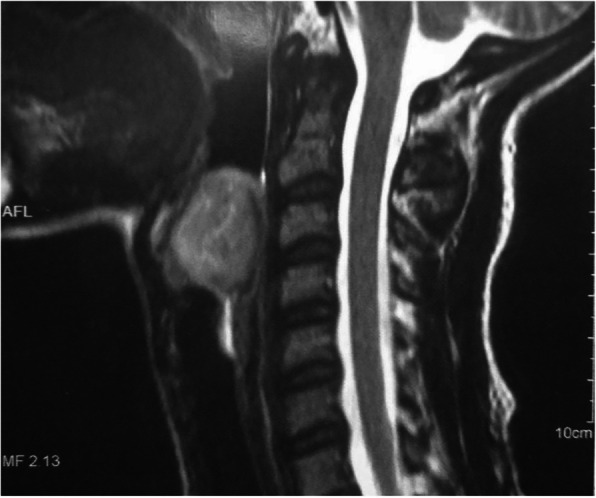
Magnetic resonance imaging (MRI) showing a well-circumscribed, clearly outlined supraglottic tumor with regular edges and hyperintensity in T2-weighted images, causing significant obstruction of the laryngeal lumen
